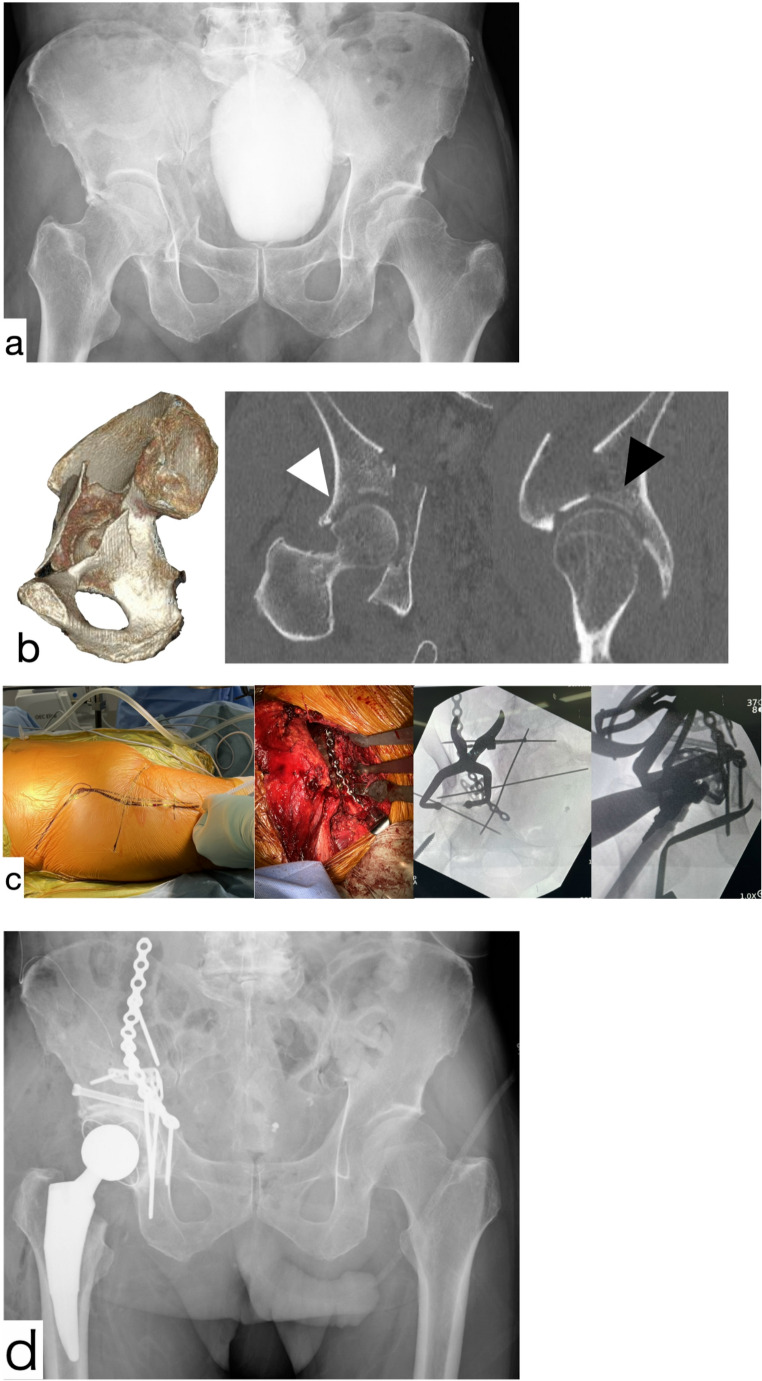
73-year-old male with anterior column fracture treated by C-THA using the iliofemoral approach AP X-ray at the time of injury. Dome impaction and femoral head injury existed CT scan at the time of injury. 3D CT shows the anterior column and isolated QLS fractures. Coronal view shows femoral head impaction (white arrow). The sagittal view shows a dome impaction fragment (black arrow) Intraoperative findings show skin incision, plate fixation through iliofemoral approach, and intraoperative image views AP X-ray just after the operation (C-THA). HHS at 3 months was 79
